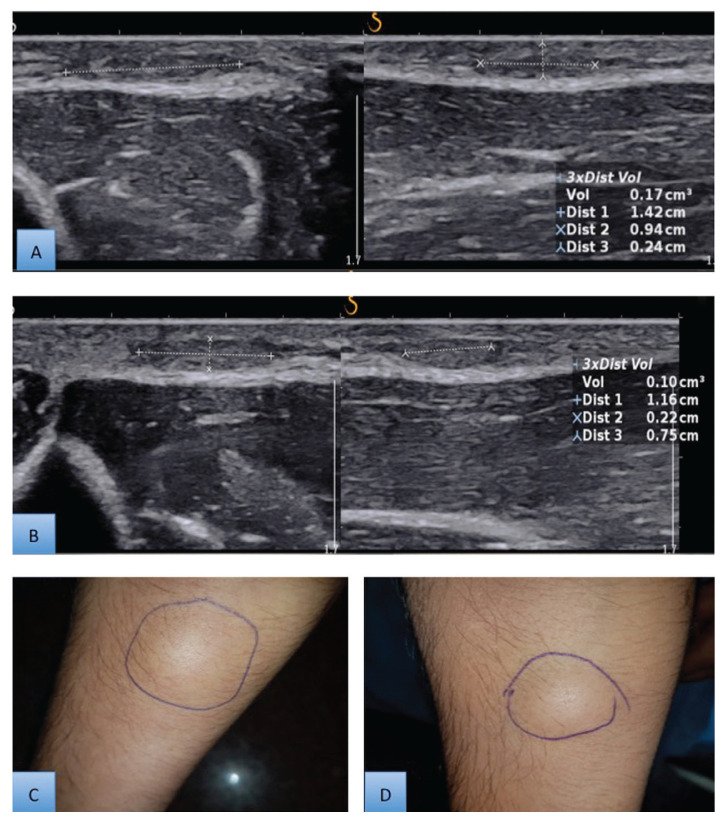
Measurement of response in mesotherapy group on ultrasonography. A. Pre-treatment dimensions of lipoma on ultrasonography; B. Post-treatment dimensions of lipoma on ultrasonography; C. Pre-treatment appearance of lipoma on inspection; D. Post-treatment appearance of lipoma on inspection
